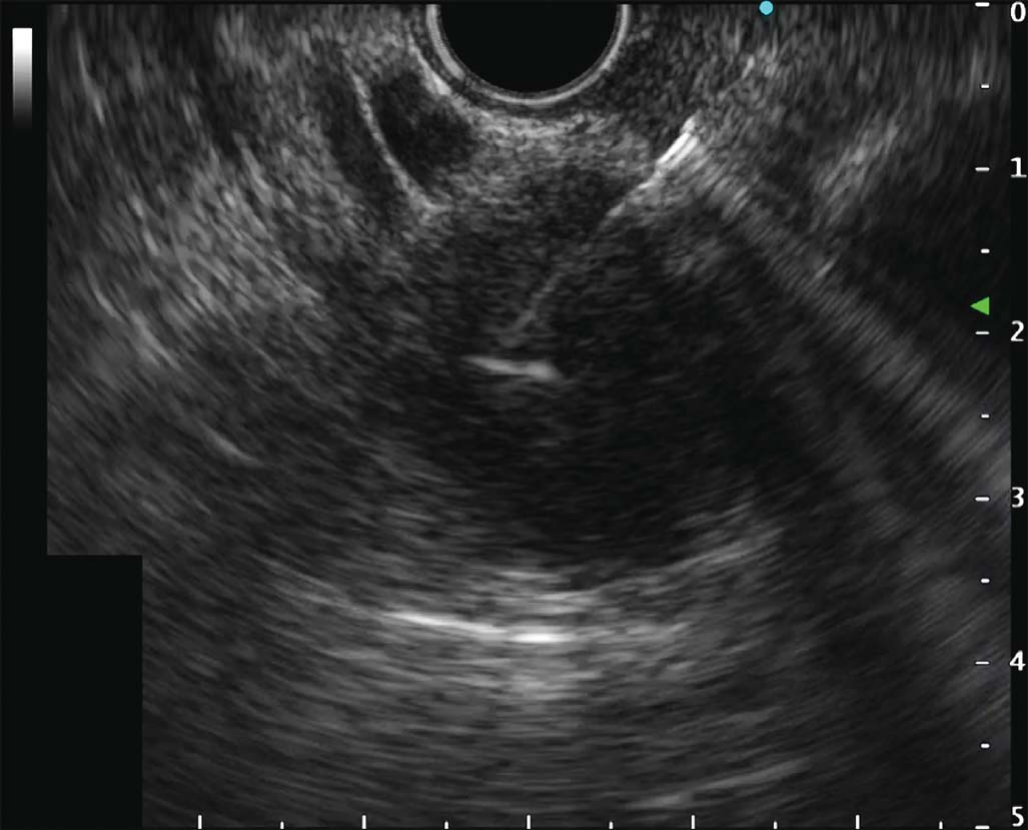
Case 1: Pancreatic adenocarcinoma in the pancreatic tail. Endoscopic ultrasound–guided fine-needle aspiration (EUS-FNA) was performed.
